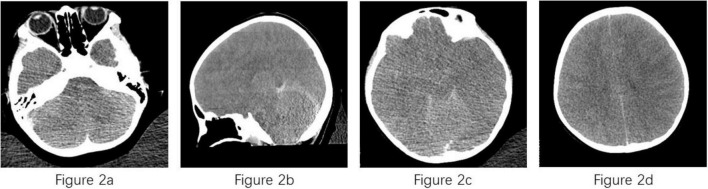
Computed tomography of case. (a) Diffuse swelling on both sides of brain; (b) unclear outline of the brainstem; (c,d) loss of brain sulcus, cistern and ventricles.
